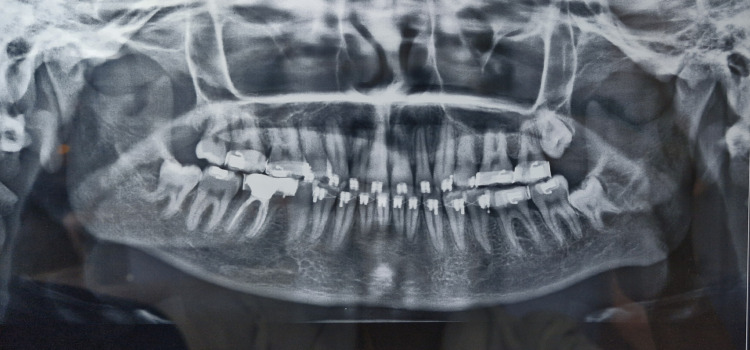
Post-treatment orthopantomogram.
